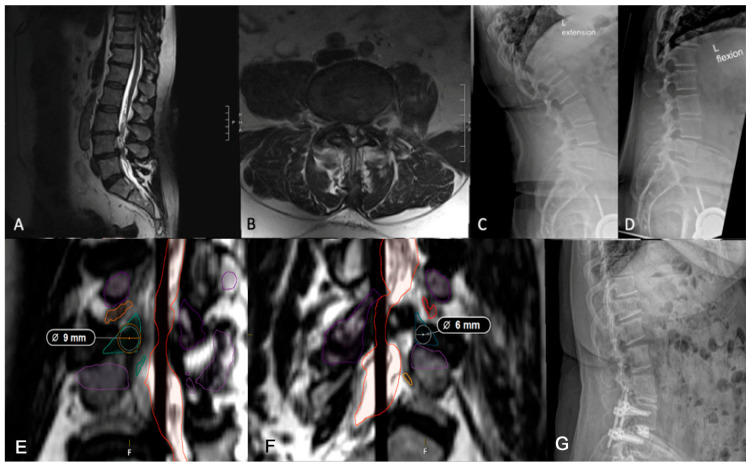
(A,B) Preoperative lumbar MRI demonstrating L4/5 spondylolisthesis. It should be noted that the patient did not complain of a radiculopathy, despite a small-sided left facet cyst at L4/5. (C,D) Preoperative flexion and extension radiographs assessing dynamic instability showing grade I spondylolisthesis at L4/5. (E) Axial imaging showing a right-sided working corridor measuring 9 mm. (F) Left-sided corridor measuring 6 mm, supporting a right-sided approach. (G) Postoperative lumbar radiograph demonstrating appropriate implant placement.
